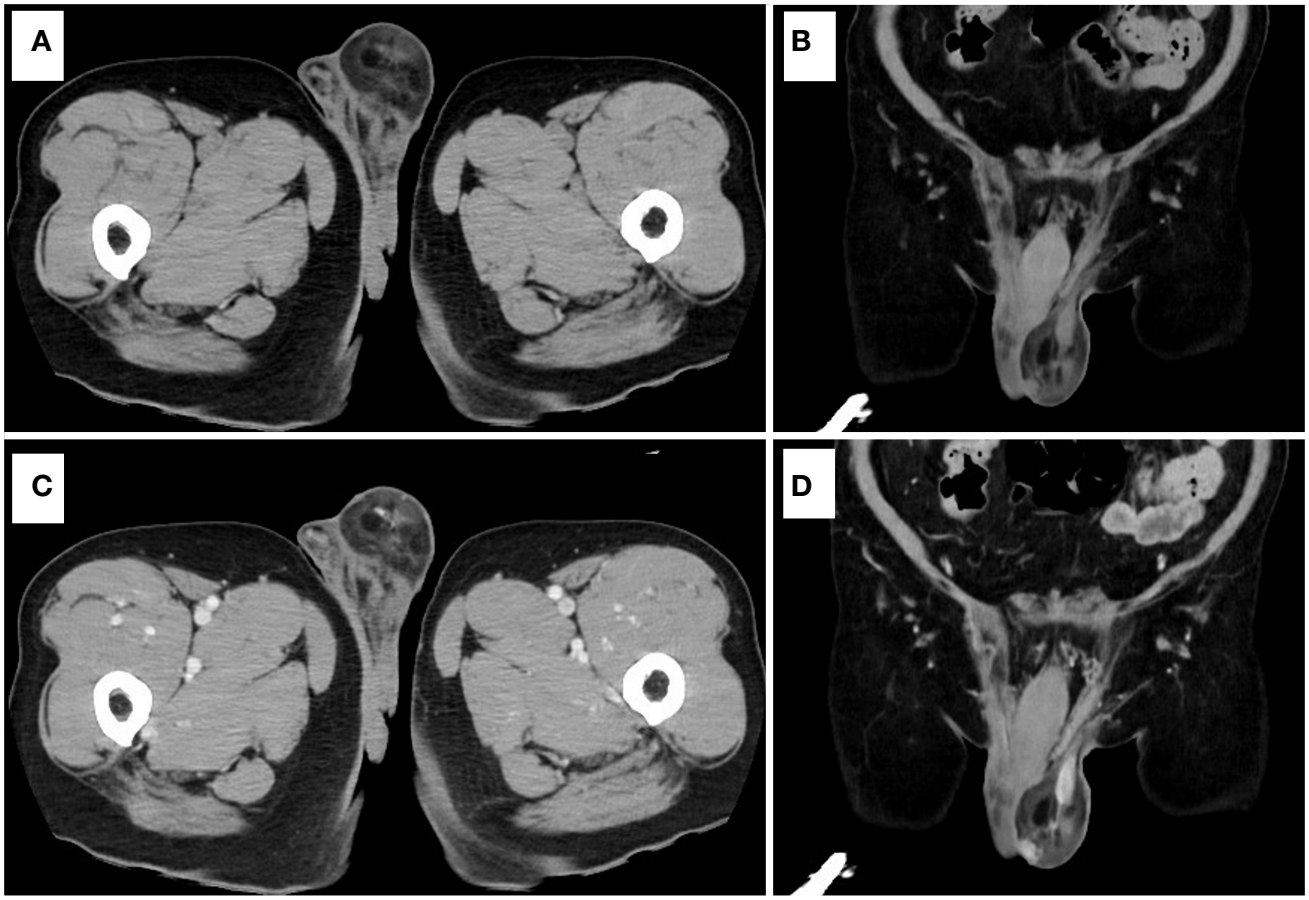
Contrast-enhanced CT. (A) Plain CT (axial view). (B) Plain CT (coronal view). (C) Contrast-enhanced CT (axial view). (D) Contrast-enhanced CT (coronal view).
